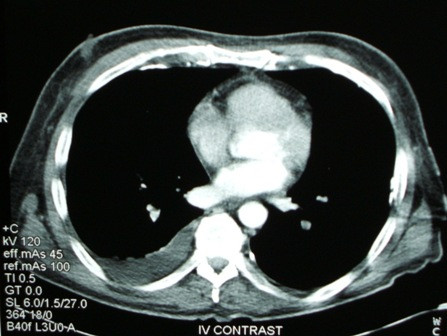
Computed tomography scan showing right pleural effusion.
